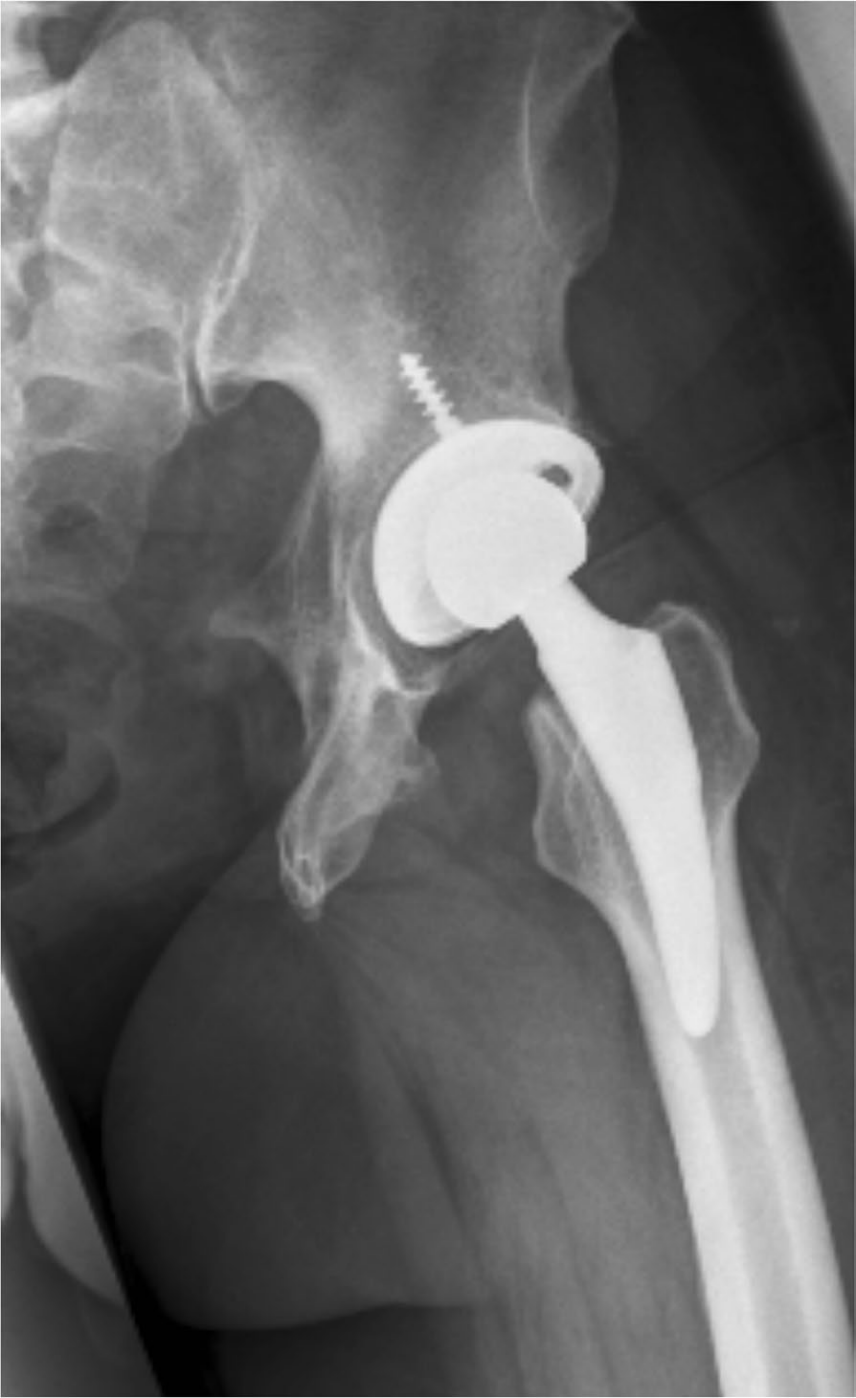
5-days postoperative antero-posterior radiography of the pelvis which shows conventional cup (Allofit) with a metaphyseal fitted stem (Fitmore)
